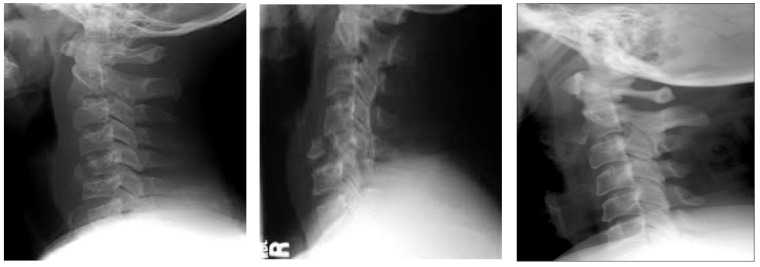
X-ray images for the fractured CS.
